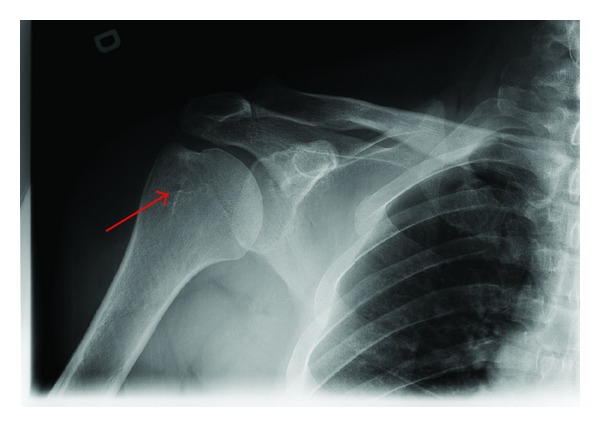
Plain radiograph of right shoulder showing small degenerative cysts at the site of insertion of the supraspinatus tendon on the greater tuberosity (arrow), indicative of rotator cuff tendinopathy.
